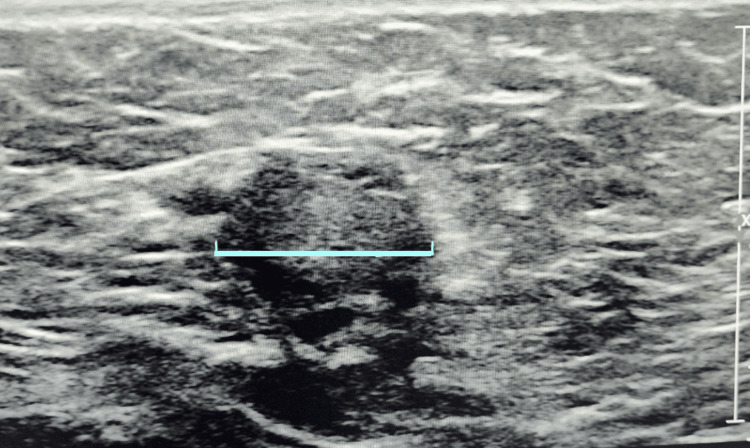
Left testicle ultrasound. Blue line showing the length of the left testicle.
